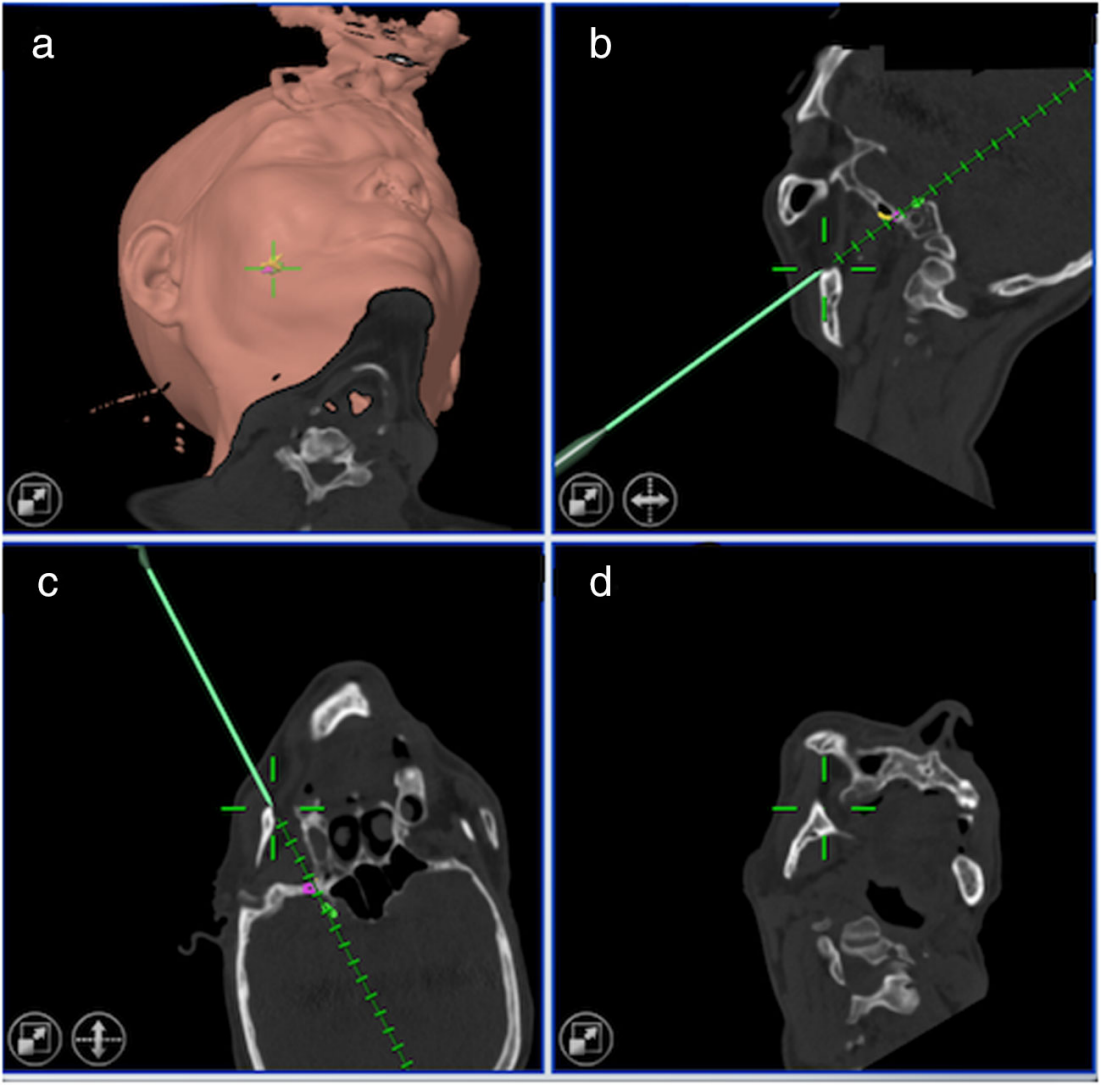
The intraoperative images of a 68-year-old man who underwent RF-TR by the use of the intraoperative CT with MRI fusion-guided technique. The trajectory (green line) was chosen along an unobstructed linear pathway to penetrate foramen ovale (purple circle) into the trigeminal cistern (green circle). The lateral pterygoid plate (yellow line) should be avoided during penetration. Three-dimentional skin probe eye view (a), inline sagittal view (b), inline axial view (c), and probe eye view (d)
